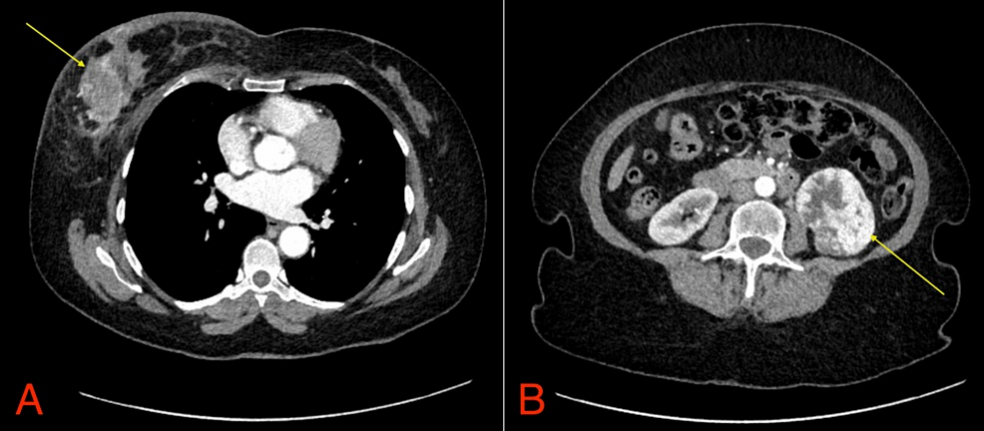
Staging CT-TAP following diagnosis of right-sided breast cancer. (A) Demonstration of right breast cancer; (B) Demonstration of left renal mass CT-TAP: CT of the thorax, abdomen, and pelvis
